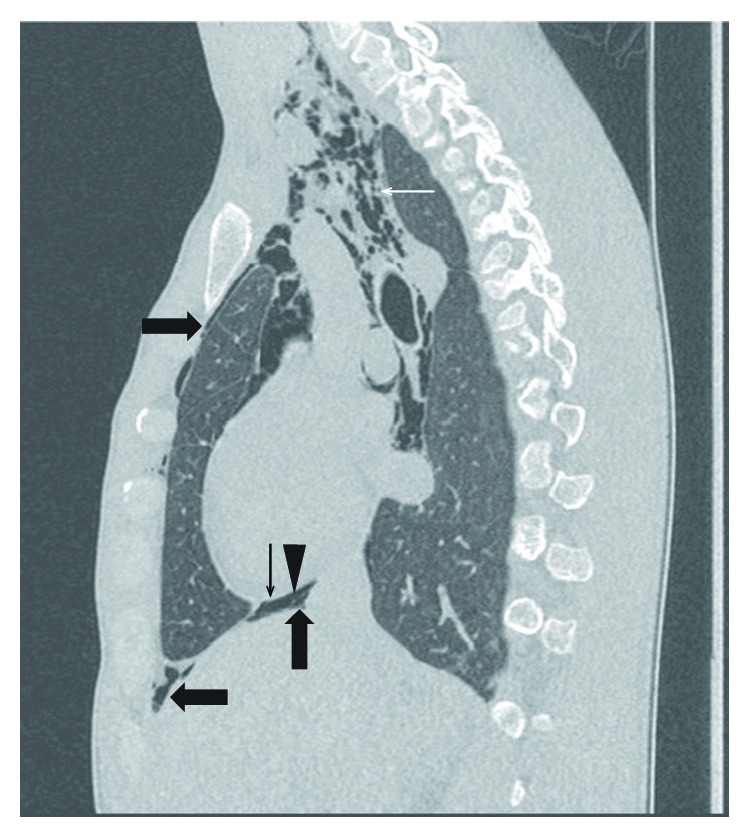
Chest CT showing extensive subcutaneous and mediastinal emphysema compatible with a large pneumomediastinum (white arrow), a pneumopericardium (thin black arrow, with pericardium marked by the black triangle), and a small pneumothorax (thick black arrows).
